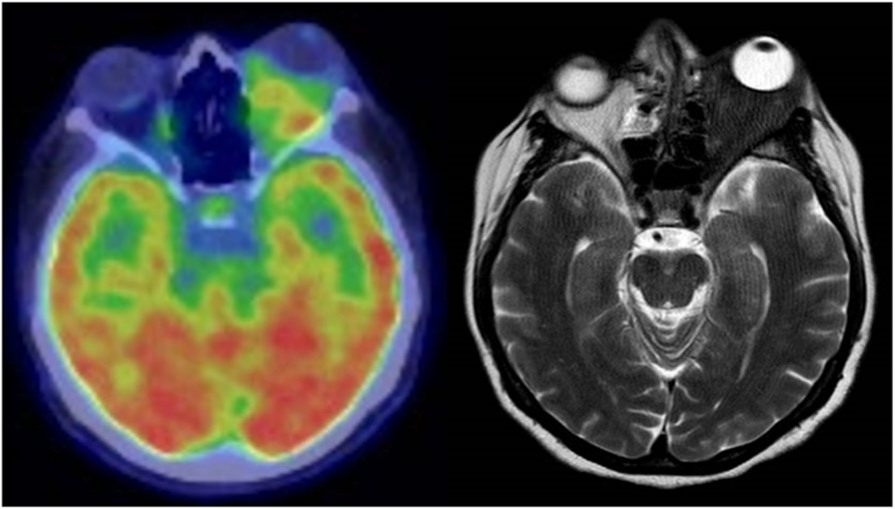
a) ^18F^FDG-PET/CT (patient 18 of Table 1) shows an abnormally increased FDG uptake and b) MR T2-weighted in transaxial section reveals a large mass at the level of the left orbital cavity and in the retrobulbar area that caused marked proptosis
